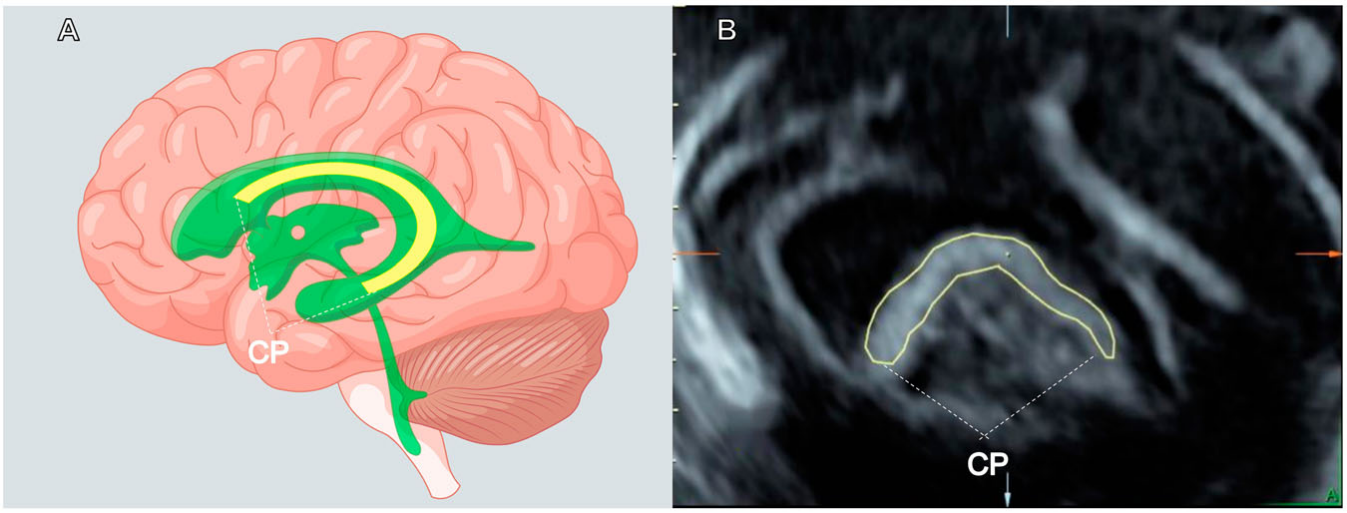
Determination of section for CP assessment (A By Figdraw; B By ultrasound), the three-dimensional images of fetal CP were acquired from the parasagittal plane of the fetal head
